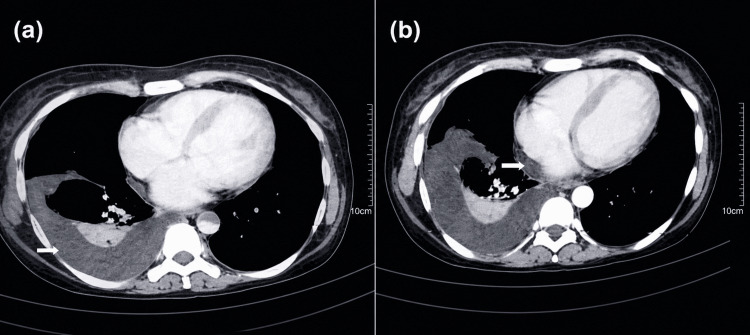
CECT of the thorax demonstrating disseminated involvement: (a) right-sided pleural effusion (arrow) and (b) small pericardial effusion (arrow) CECT images demonstrating right-sided pleural effusion and associated pericardial effusion, along with bilateral ground-glass opacities and mediastinal lymphadenopathy. Arrows indicate the areas of interest. CECT: contrast-enhanced computed tomography
